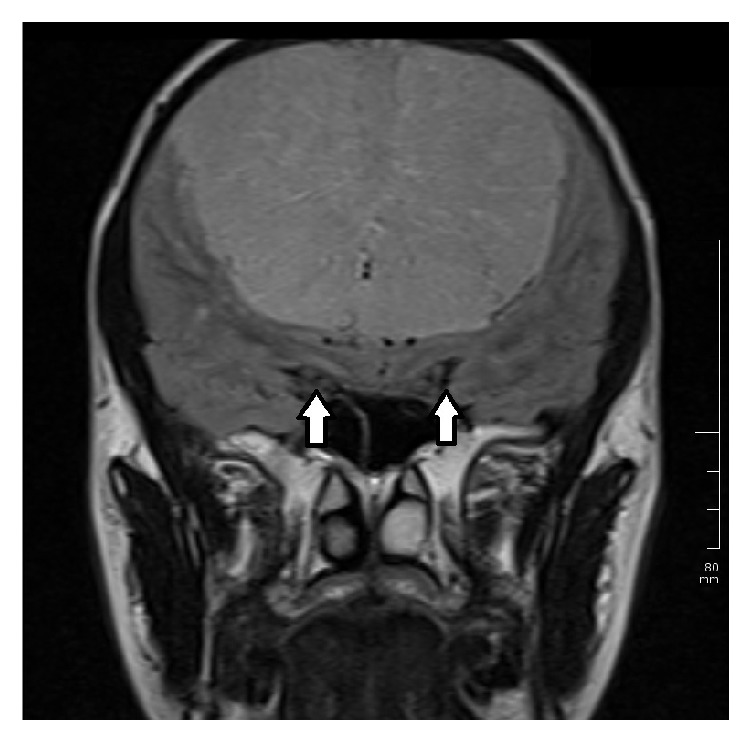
Magnetic resonance imaging. Coronal T2-weighted images show that the mass was exerting pressure on the chiasm.
